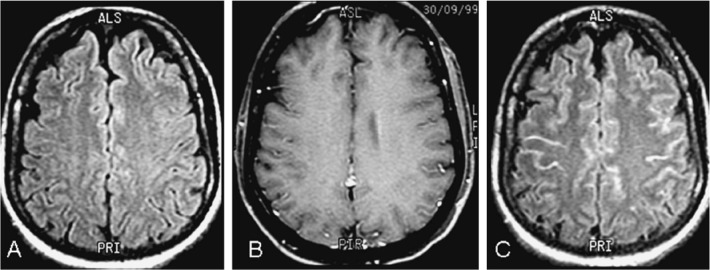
Patient with CSF analysis positive for meningitis A: Axial FLAIR imaging without contrast shows no abnormality. B: Axial post-contrast T1W1 without leptomeningeal enhancement. C: Axial post-contrast FLAIR imaging. Note in this example how this sequence shows significant leptomeningeal enhancement (arrows) comparing to the T1W1 (Ferreira et al., 2005).
